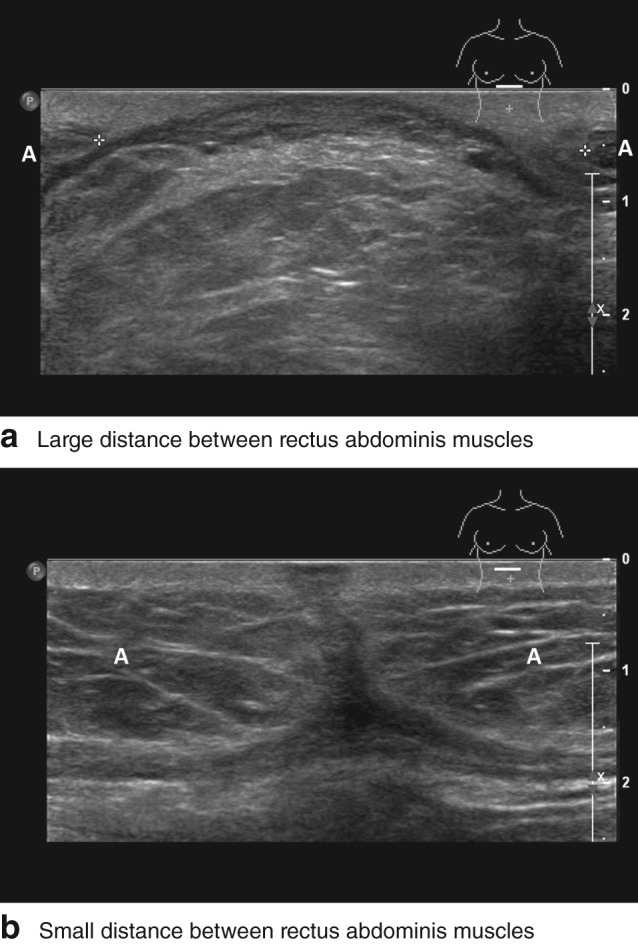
Ultrasound images at 1 month after surgery from a 63‐year‐old man with a median laparotomy scar from xiphoid to umbilicus. a At the upper one‐third level of the laparotomy scar, bulging of intra‐abdominal fatty tissue through a large distance of 4·3 cm between the medial borders (indicated by + markers) of the rectus abdominis muscles can be seen. The patient developed an incisional hernia during follow‐up. b At the two‐thirds level of the laparotomy scar, a tight junction is visible between the medial borders of the rectus abdominis muscles in the midline. a,b The body mark (upper left) indicates the level and position (axially oriented) of the ultrasound probe (10–12 MHz, linear array transducer). A, rectus abdominis muscle
